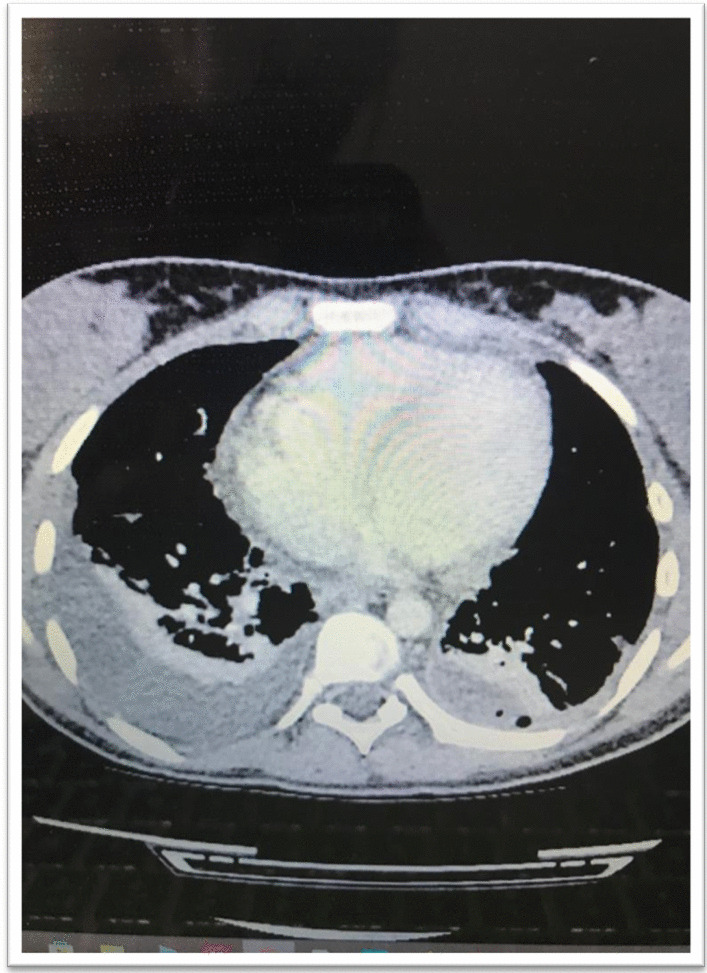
Thoracic computed tomography, mediastinal window: pleural fluid with dense content
